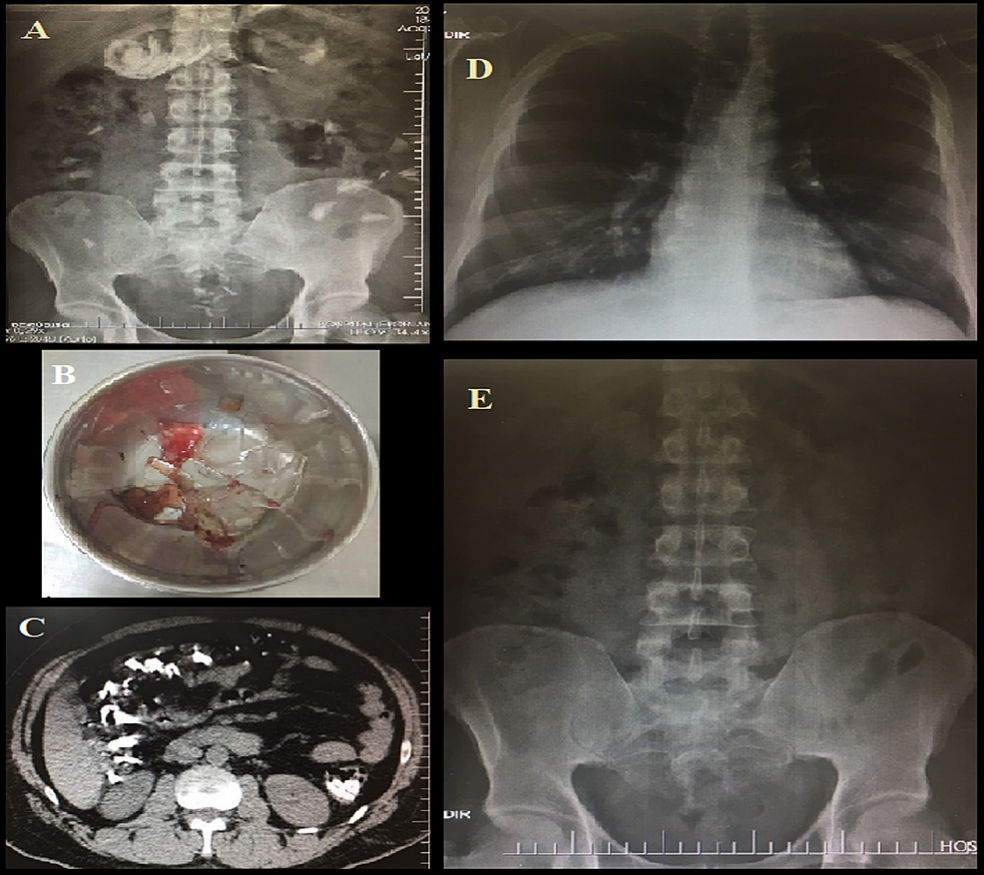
Radiographic and upper gastrointestinal endoscopy findings Panel A: radiograph of an orthostatic abdomen (first hospitalization day) showing several radiopaque fragments (glass) inside the stomach and in the intestine. Panel B: metal container holding several glass shards that were removed from the patient's stomach through upper gastrointestinal endoscopy. Panel C: total abdomen tomography (without intravenous contrast) performed for control purposes (second hospitalization day) showing glass shards inside the intestine, although with no signs of pneumoperitoneum. Panels D-E: radiological control performed on the last hospitalization day showing the complete elimination of the glass shards and lack of pathological intracavitary air signs.
